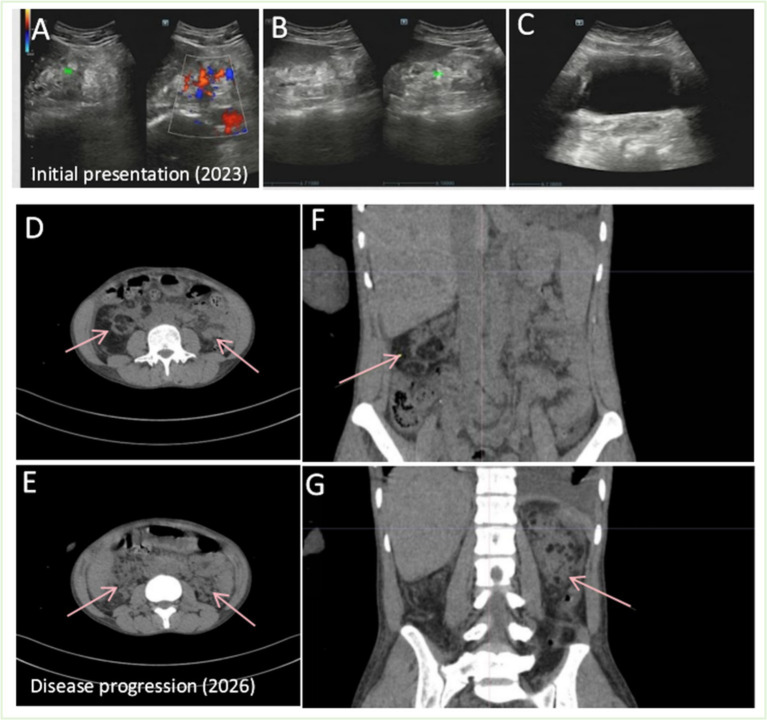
Evolution of renal imaging findings from 2023 to 2026. (A–C) Renal ultrasound obtained at initial presentation in 2023 demonstrating increased renal parenchymal echogenicity without evidence of significant angiomyolipomas or large space-occupying renal lesions. (D,E) Axial and (F,G) coronal abdominal CT images obtained in 2026 showing marked bilateral renal enlargement with numerous fat-density nodules consistent with diffuse angiomyolipomas. Mild perinephric fat stranding is present at the mid-to-lower poles. Arrows in (F,G) indicate the remaining renal parenchyma, suggesting extensive renal involvement.
